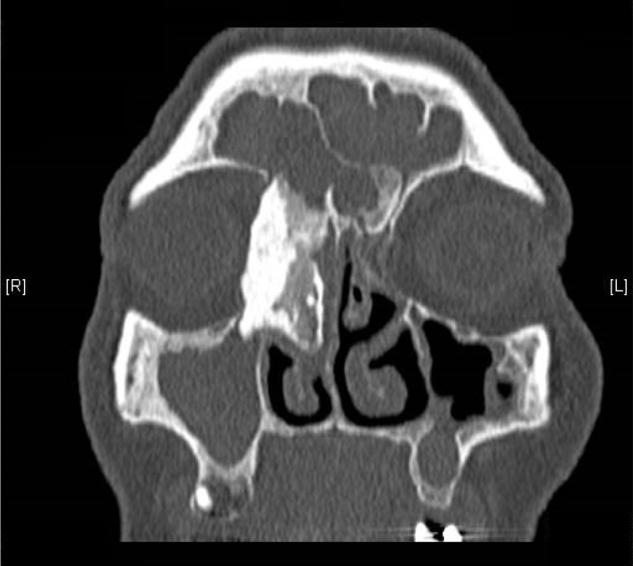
CT scan of the sinuses – fronto-ethmoidal osteoma sized about 39.5 × 19.8 × 19.4 mm, coronal plane.
